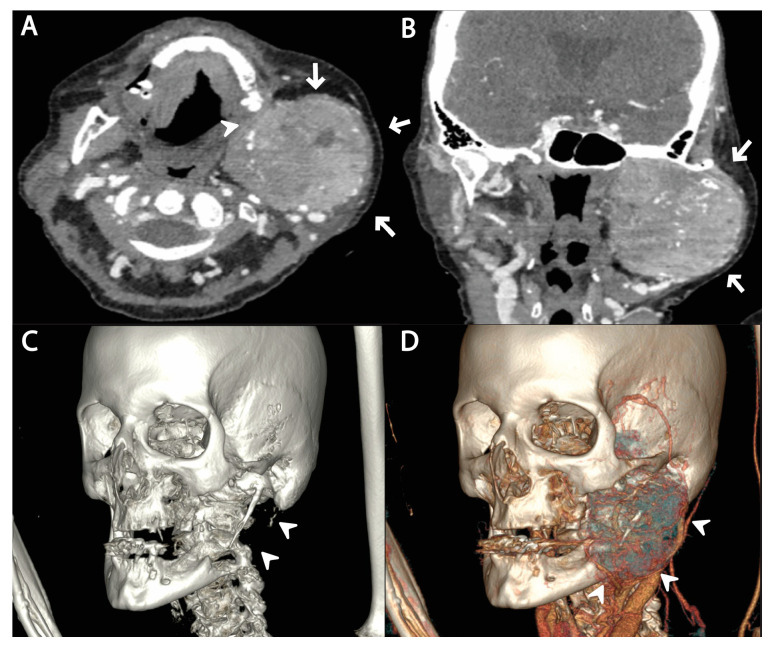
Axial (A) and sagittal (B) images of contrast-enhanced neck CT scan show a large, lobulated and intensively opacified osteolytic soft-tissue mass in the left mandible angle and ramus (arrows), extending from the base of the skull to the mandibular angle. Infiltration of masticatory muscles is observed as well. The volume-rendered images show destructed left mandibular ramus and mandible angle (arrowheads) due to tumor invasion (C), as well as an image of vascular 3D reconstruction, which shows highly vascularized tumor (arrowhead) (D).
